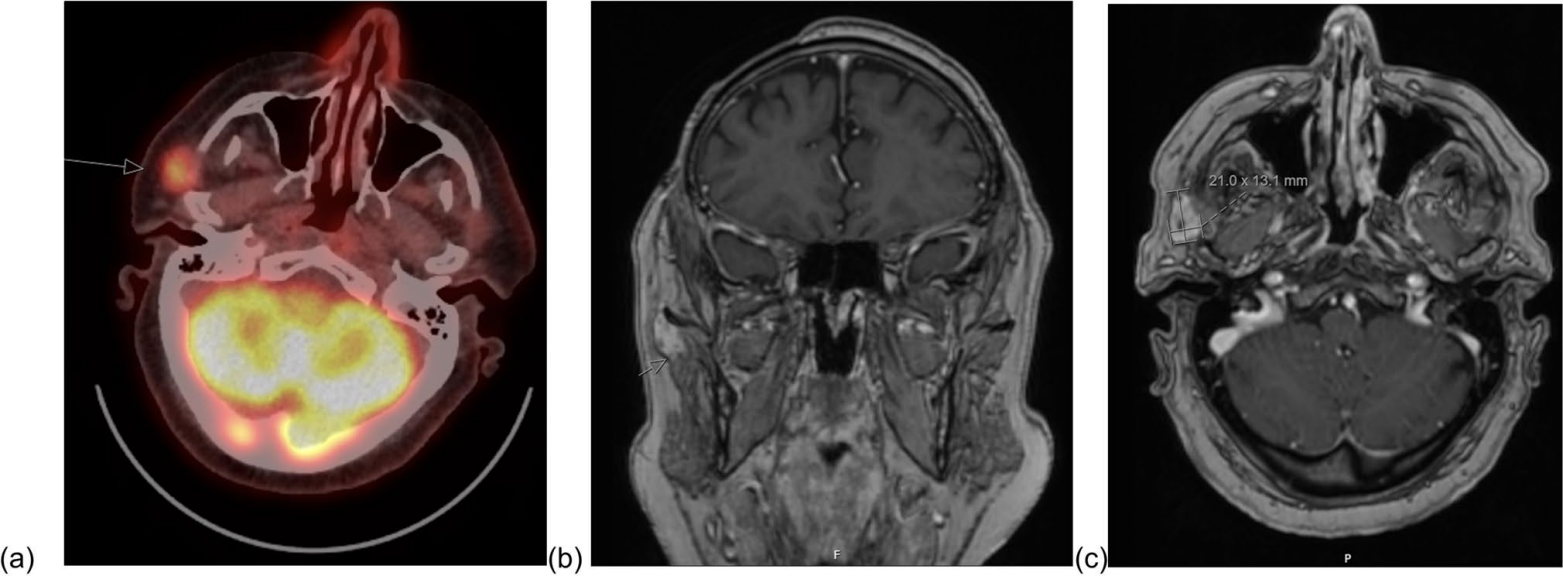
Pre-operative imaging a Fused Axial Positron Emission Tomography-Computed Tomography (PET-CT), b and c magnetic resonance imaging (MRI) of the head with contrast
